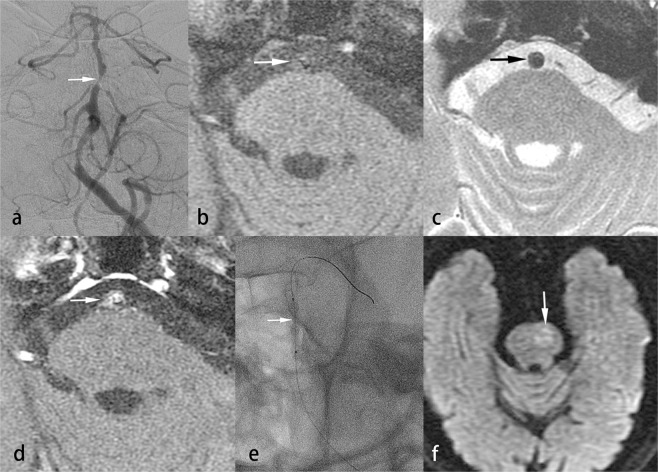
Hard lesion in a patient with atherosclerosis. A 73-year-old man suffered from recurrent stroke in the bilateral occipital regions. Left vertebral angiogram showed atherosclerosis with 90% stenosis of the mid-basilar artery (a, arrow). VW-MRI of the stenotic lesion of the mid-basilar artery revealed low signal on fat-suppressed T1WI (b, arrow) and T2WI (c, arrow) and strong enhancement on contrast-enhanced fat-suppressed T1WI (d, arrow). The PTAS failed because the stenotic lesion could not be fully dilated with the PP up to 7 atm (e, arrow). The Wingspan stent system could not pass through the stenotic lesion. His case was also complicated by a perforator infarct in the left pons (f, arrow).
